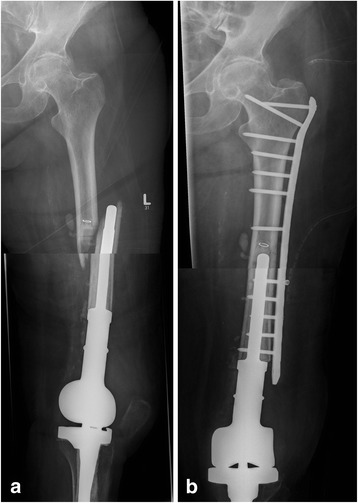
X-ray images of an 82-year-old woman with a periprosthetic fracture of the/left femur with distal femoral replacement; (Type D II) a: anterior-posterior radiation path with representation of the fracture before supply; b: anterior-posterior radiation path after reconstruction and supply by plate osteosynthesis
